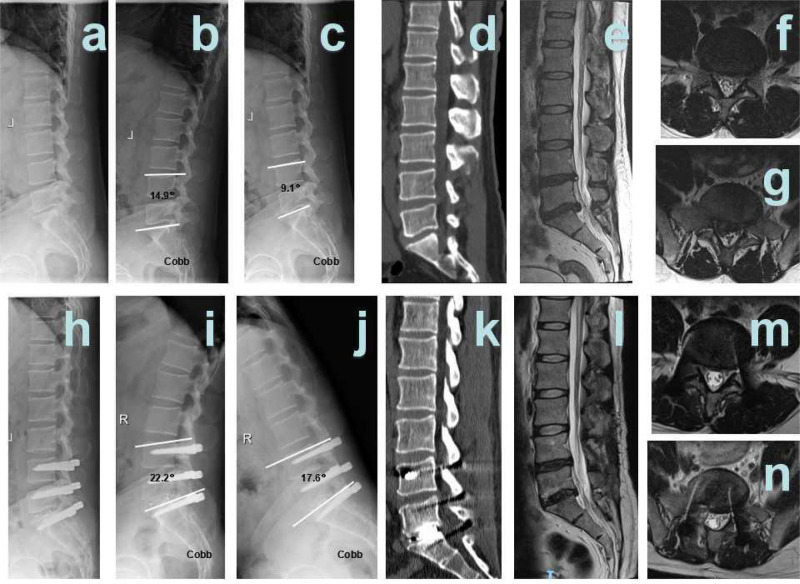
Hybrid patient 2. (A) Pre-surgical lateral X-ray; (B) pre-surgical hyper-extension X-ray; (C) pre-surgical hyper-flexion X-ray; (D) pre-surgical CT showed L5 I° spondylolisthesis; (E) pre-surgical T2 weighted MRI sagittal plane showed L4/5, L5/S1 disc herniation; (F) pre-surgical MRI showed L4/5 disc herniation and spinal stenosis; (G) pre-surgical MRI showed L5/S1 disc herniation and spinal stenosis; (H) 12 months after surgery, Post-surgical lateral X-ray; (I) 12 months after surgery, post-surgical hyper-extension X-ray; (J) 12 months after surgery, post-surgical hyper-flexion X-ray; (K) 12 months after surgery, post-surgical CT showed interbody fusion; (L) 12 months after surgery, post-surgical T2 weighted MRI sagittal plane; (M) 12 months after surgery, post-surgical MRI sagittal plane showed that L4/5 spinal stenosis was improved significantly; and (N) 12 months after surgery, post-surgical MRI sagittal plane showed that L5/S1 spinal stenosis was improved significantly.
